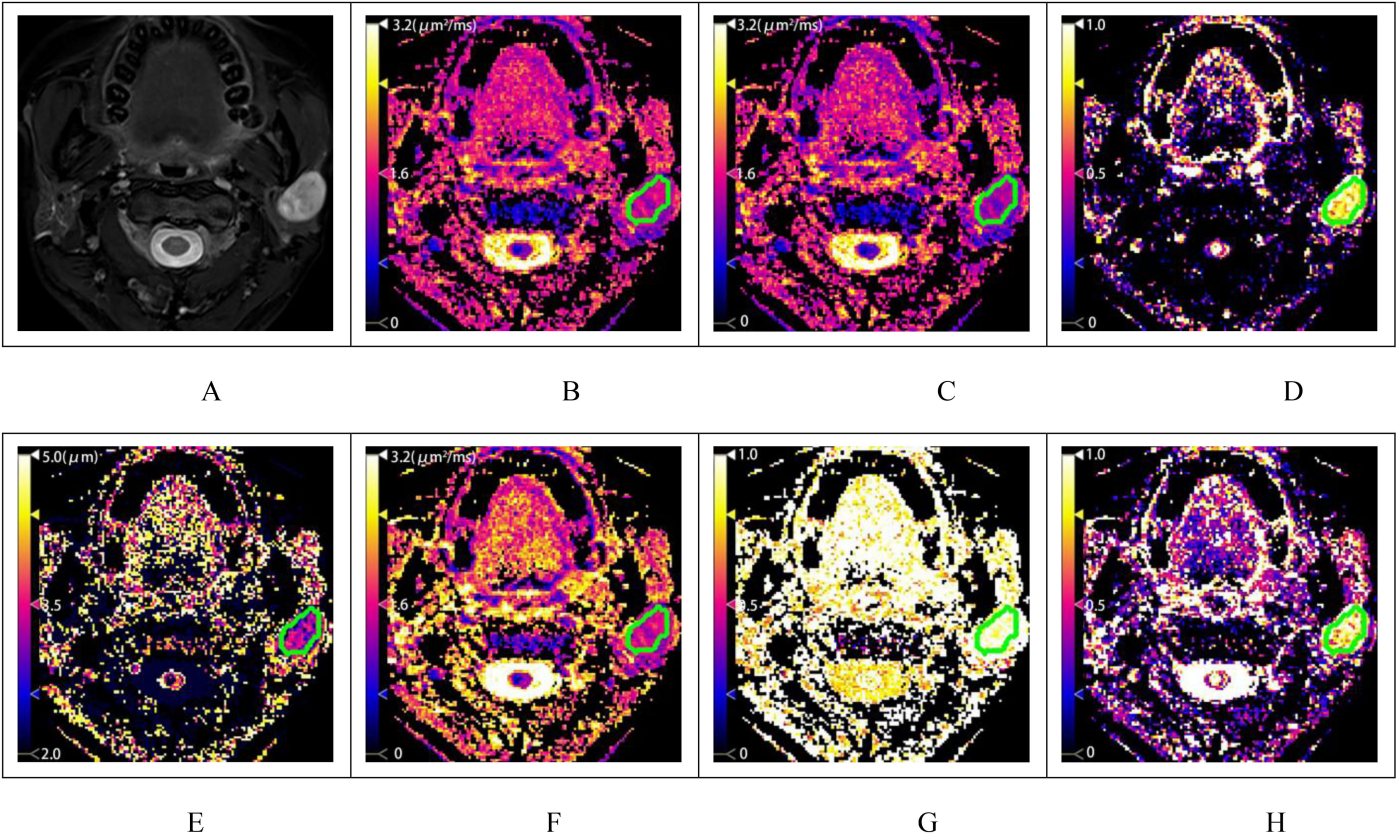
A patient with pleomorphic adenoma of the left parotid gland. (A) shows the T2-weighted fat-saturation image, and (B–H) display the diffusion-weighted image (DWI), fractional anisotropy (FROC), and color maps of the CTRW model parameters, respectively. The measured values are as follows: ADC=1.510μm^2^/ms (2B), D_FROC_=1.203μm^2^/ms (C), β_FROC_=0.888 (D), μ_FROC_=3.021μm (E), D_CTRW_=1.412μm^2^/ms (F), α_CTRW_=0.971 (G) and β_CTRW_=0.891 (H).
